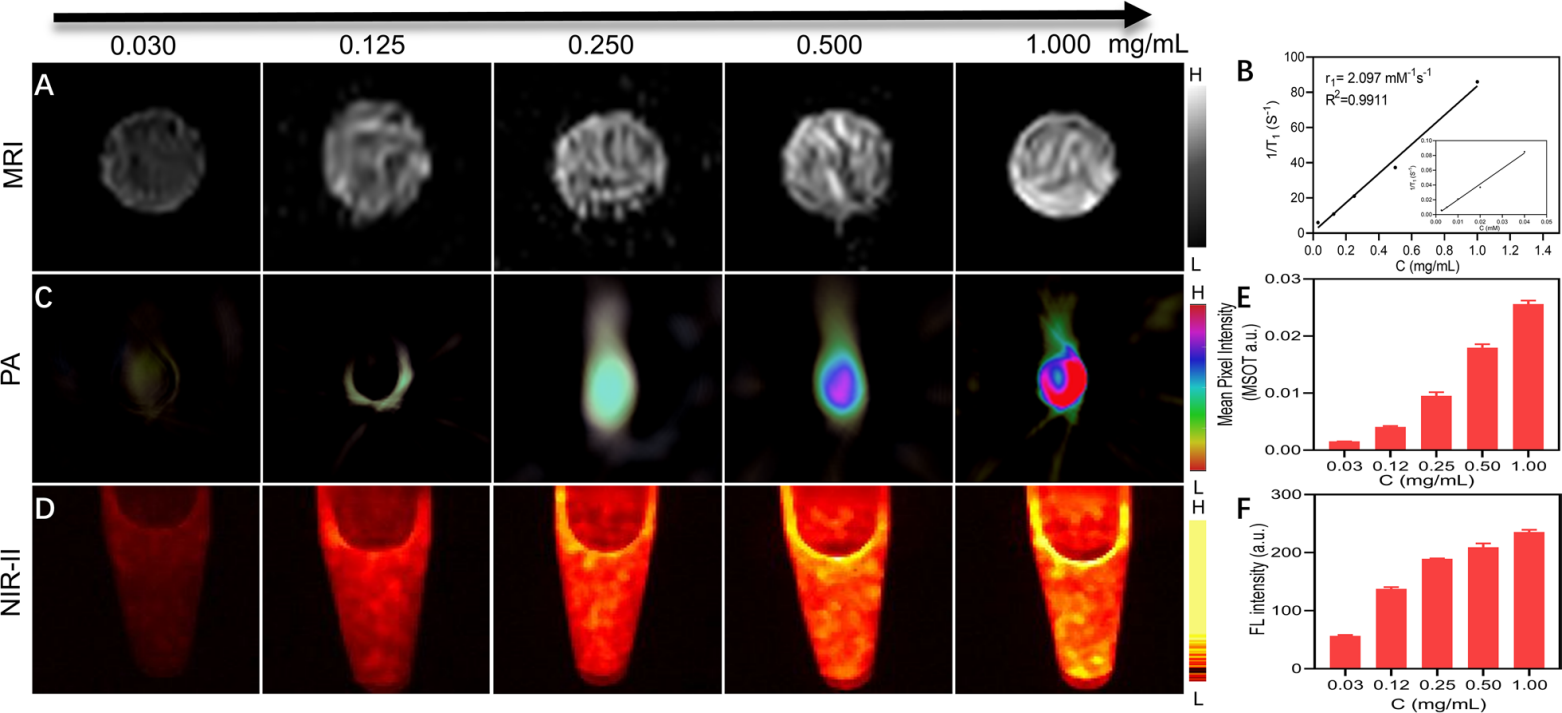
The multimodal imaging performance of IFDG. A MRI images of IFDG at different concentrations. B T1 relaxation rate curve of IFDG. C PA and D NIR-II images of IFDG at different concentrations. E PA and F NIR-II quantification statistics of IFDG
